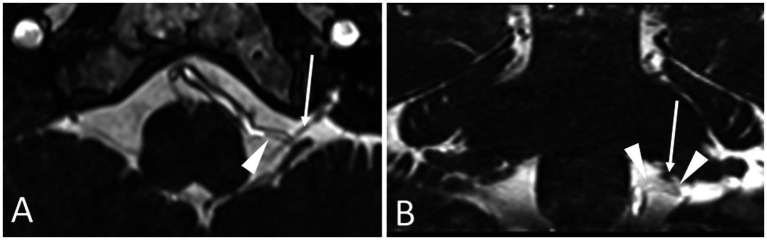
Axial (A) and coronal (B) MRI showing the PICA (arrowhead) encroaching the vagus nerve (arrow).
